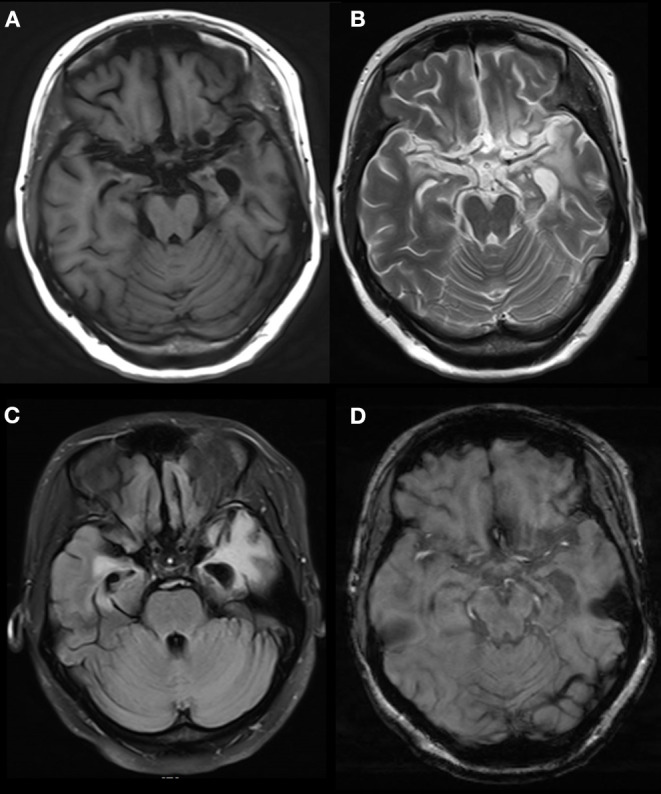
Abnormal signals in bilateral temporal, insular and hippocampal regions indicated by brain MRI. MRI showed a long T2 signal in bilateral temporal, insular and hippocampal slices, with a fuzzy border and uneven signals on the left side. A FLAIR scan showed a strong signal, and no obvious abnormal enhancement was found after injection. The temporal angle of the left lateral ventricle was enlarged, and the midline remained in the middle. MRI, magnetic resonance imaging. (A) T1 (T1-weighted imaging). (B) T2 (T2-weighted imaging). (C) FLAIR (fluid-attenuated inversion recovery). (D) T1C (MRI enhanced scan).
